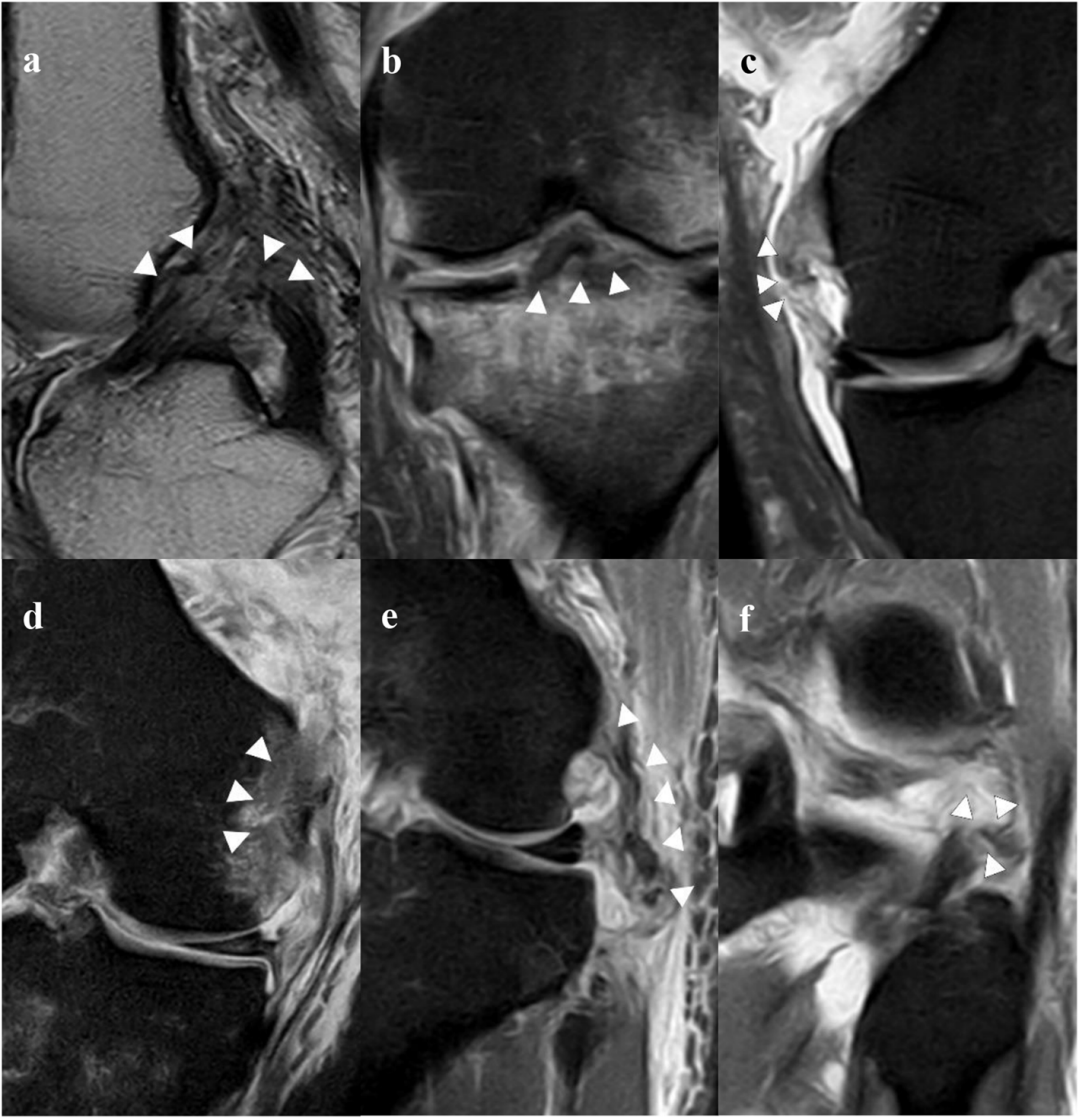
Preoperative MRI of the multiple ligaments knee injuries/knee dislocations; a The ruptured ACL and PCL; b Avulsion fracture at the ACL insertion in the tibial intercondylar eminence; c Rupture of MCL in the mid-substance; d Avulsion of MCL at the insertion of the femoral condyle; e The ruptured LCL in the mid-substance; f The ruptured popliteal tendon
